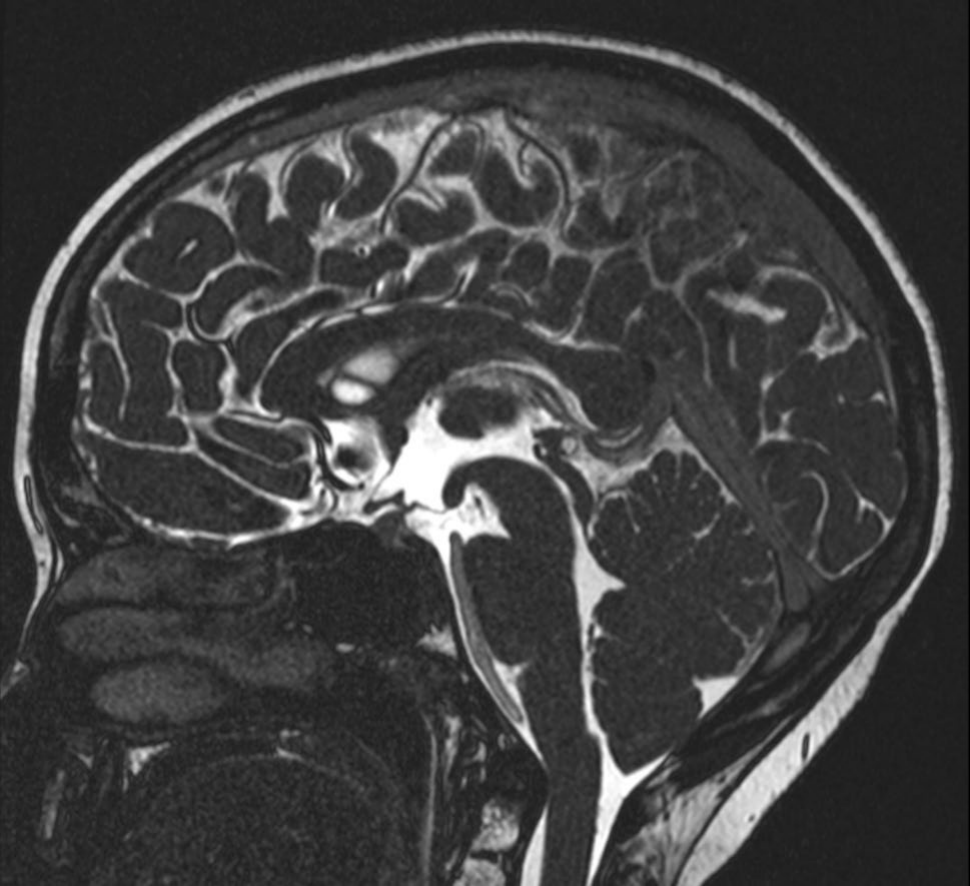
Resolution of cerebellar sagging, as demonstrated on MRI taken 2 weeks after the procedure
